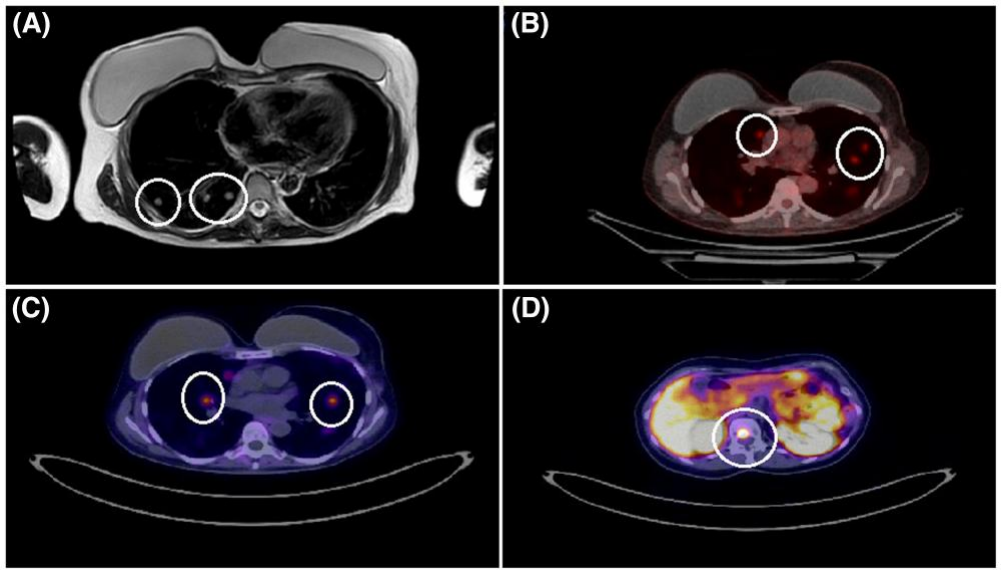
Imaging techniques were used during the case investigation. A. MRI of the abdomen (T2-weighted axial view) capturing the lower lung fields showed suspicious lesions (white circles). B. Fluorine-18 fluorodeoxyglucose PET/CT scan (axial view) depicted bilateral lung nodules (white circles). C. ^68^Ga-DOTATATE PET/CT scan (axial view) revealed somatostatin receptor-expressing lung lesions (white circles). D. SSTR-expressing tissue was also detected in the body of L1 by ^68^Ga-DOTATATE PET/CT scanning (axial view, white circle).
